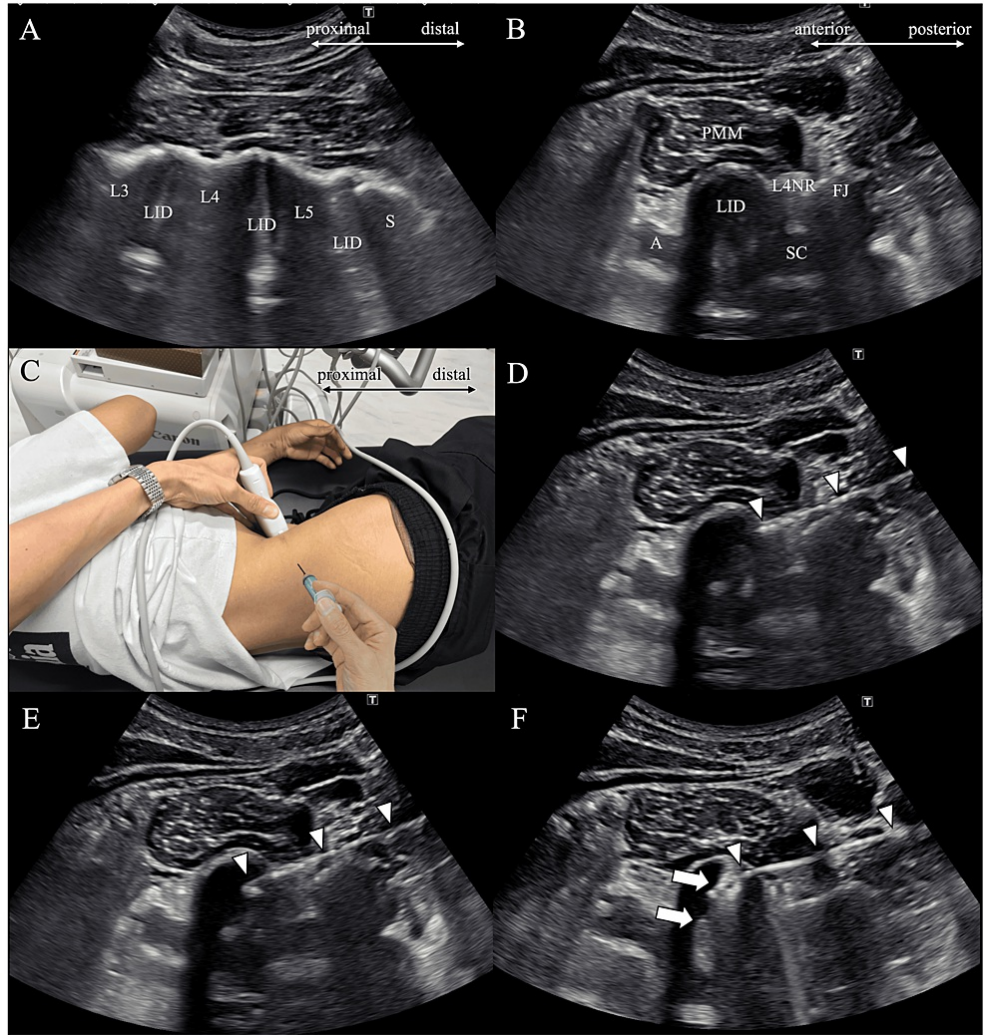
Ultrasound-guided lumbar intradiscal injection A: Identification of the lumbar vertebrae level in the long-axis view; B: Ultrasound image of the lumbar intervertebral disc and surrounding structures; C: The placement of the probe on the lumbar area and the entry point and direction of the needle; D: The needle reached the outer edge of the intervertebral disc; E: The needle tip was inserted into the intervertebral disc; F: Medication was injected into the intervertebral disc; LID: Lumbar intervertebral disc SC: spinal canal, A: aorta, PMM: psoas major muscle, FJ: facet joint, L4NR: fourth lumbar nerve root, arrowheads: needle, arrow: medication injected into the intervertebral disc
